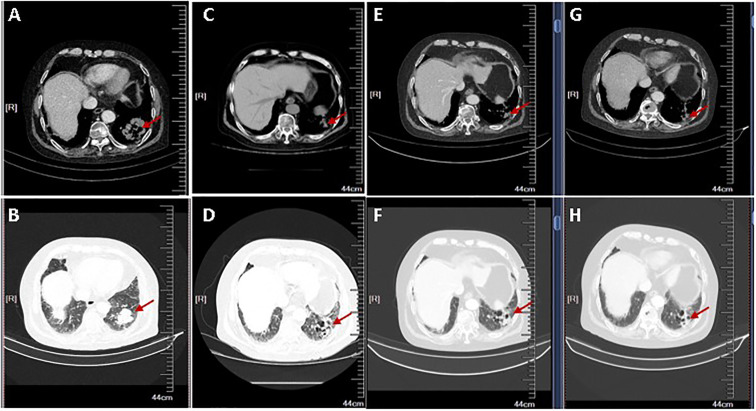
The radiological tumor evolution. Shown are two different views of chest computed tomography (CT) slices (A, C, E, G: Chest window; B, D, F, H: Lung window). (A, B) CT scan showing mass of the lung adenocarcinoma pre-treatment of PD-1 inhibitor. (C–H) CT scan shows a partial response after 2 (C, D), 4 (E, F), and 6 (G, H) cycles of immunotherapy. The arrow shows the changes of the left lung mass during the PD-1 administration.
